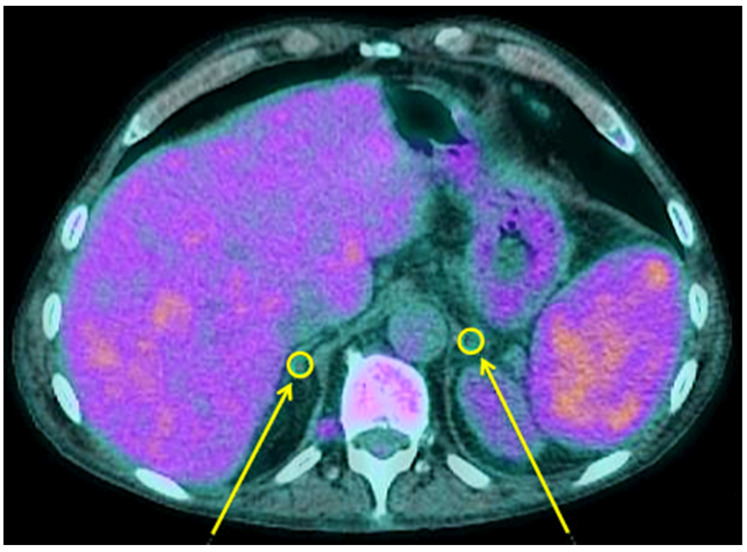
Example of calculation of quantitative parameters on adrenal glands of one COVID-19 patient. Yellow circles and arrows show the regions of interest. In this patient, the right adrenal gland had an SUVmax of 1.33 and an SUVmean of 1.21; the left adrenal gland had an SUVmax of 1.26 and an SUVmean of 1.20.
